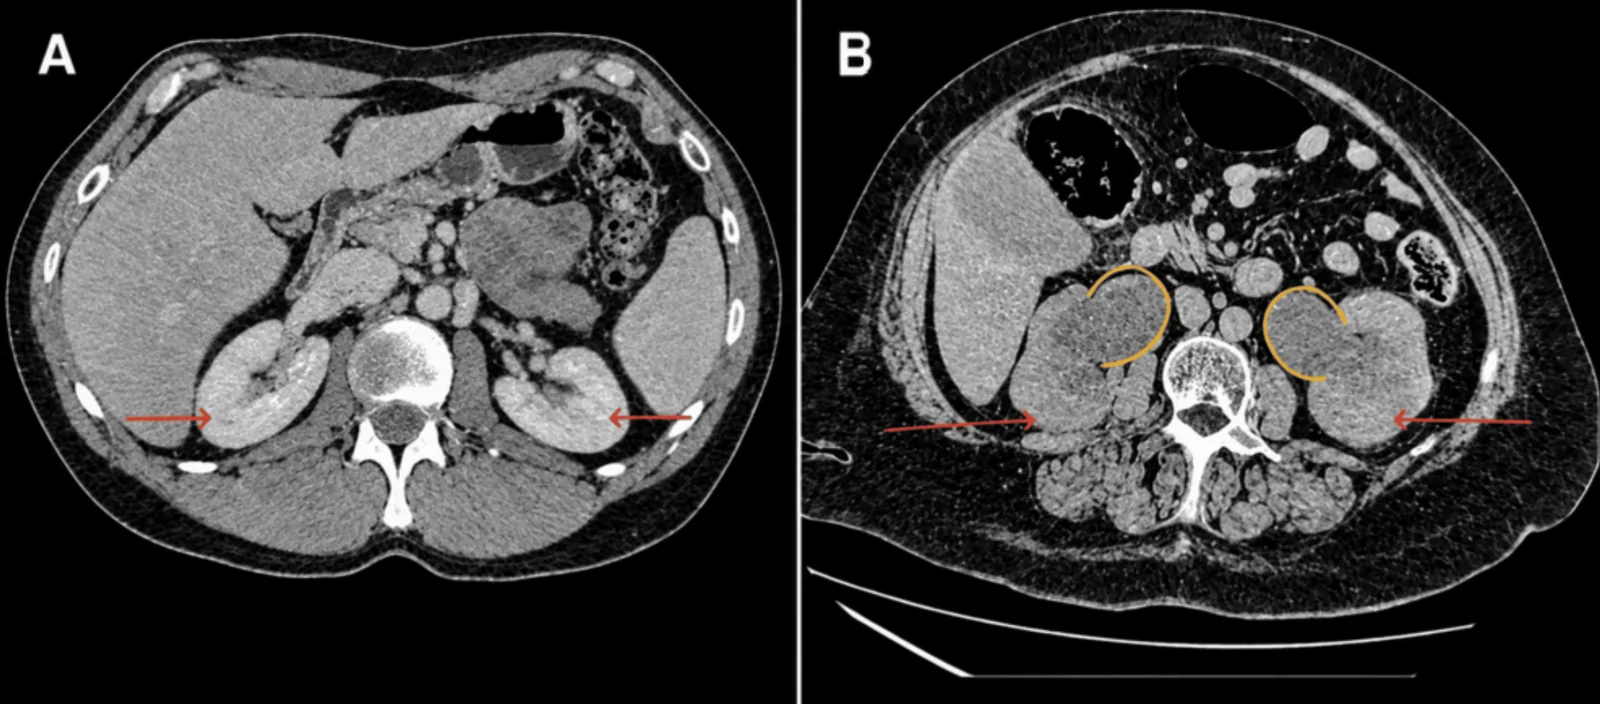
Axial view of the CT abdomen (A) Normal kidneys (red arrows) without hydronephrosis. (B) Bilateral moderate hydronephrosis (yellow line) and kidneys (red arrows) CT: computed tomography
